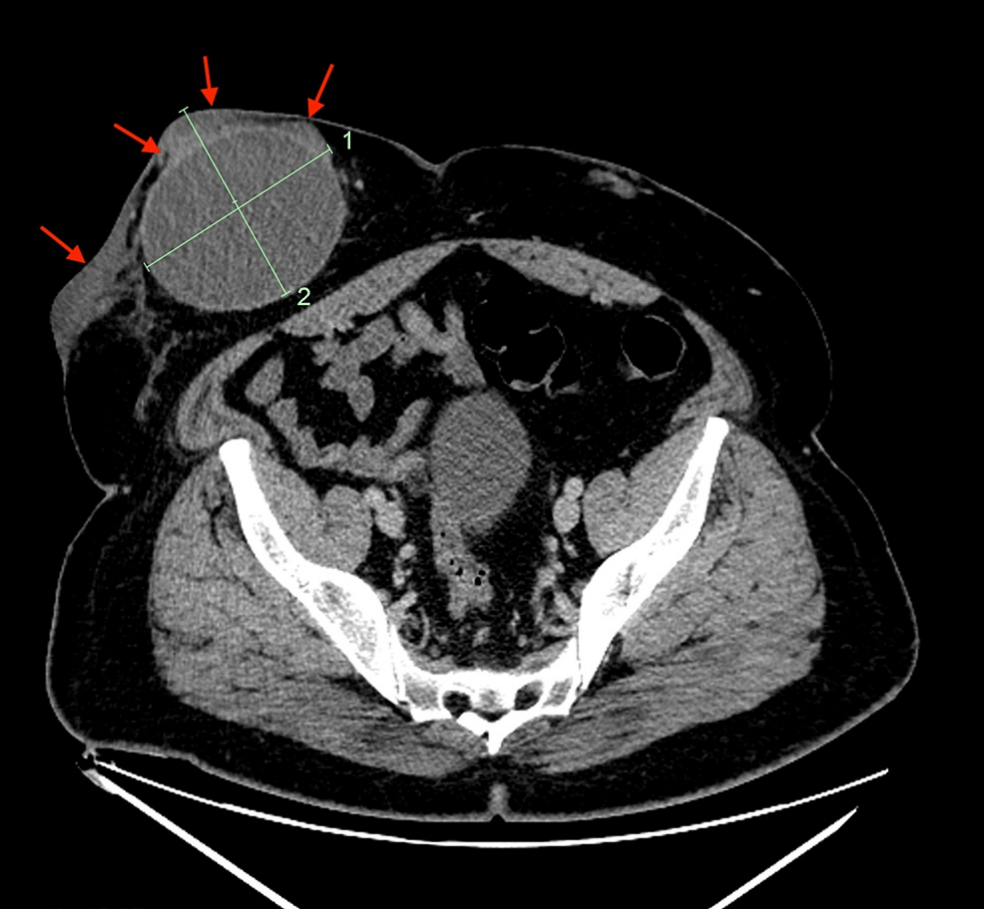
Computed tomography scan showing multiple large abdominal wall nodules with a right lower quadrant cystic mass. Computed tomography scan showing multiple large abdominal wall nodules with a right lower quadrant cystic mass measuring 10.5 × 8.8 × 10.7 cm, stable since 2017. Superficial to this cyst was skin and subcutaneous tissue thickening with fat stranding (red arrows) and a round density within the right lower quadrant cyst.
